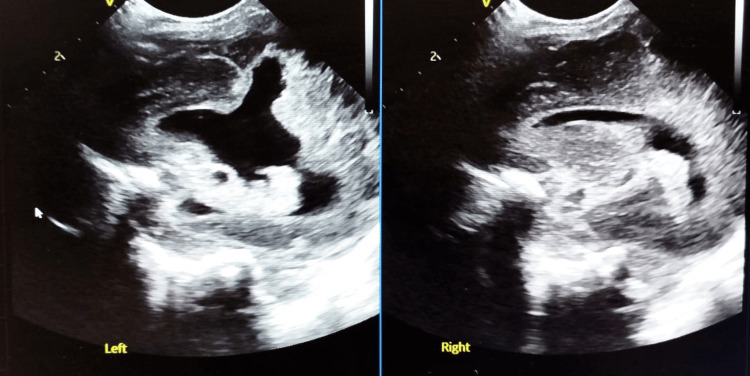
Cranial ultrasound sagittal planes Cranial ultrasound sagittal planes showing CNS malformations, with asymmetric cerebral hemispheres
